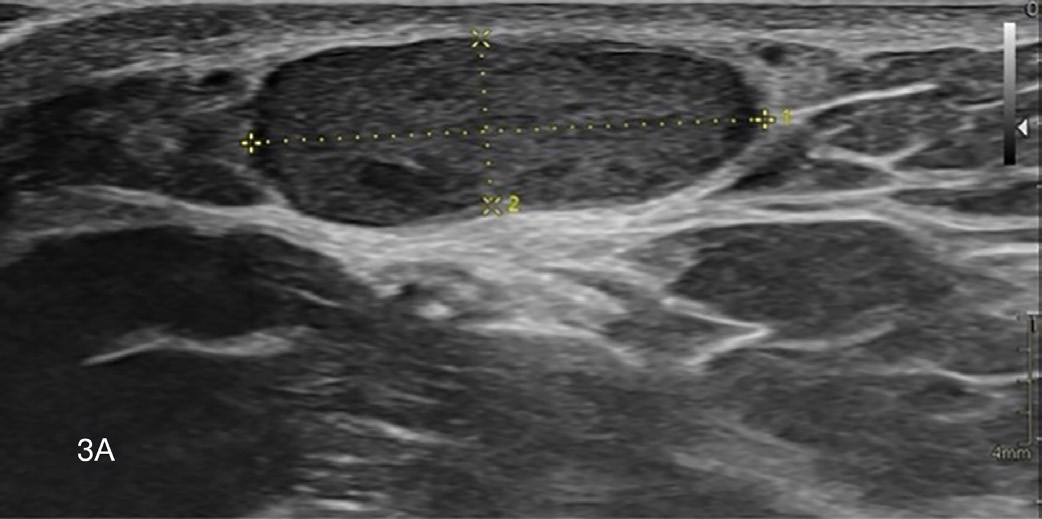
Ultrasound shows a subcutaneous hypoechoic nodule.
